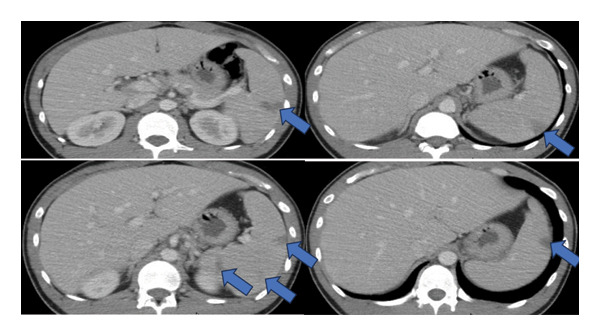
Still images from CT abdomen and pelvis from the patient’s first presentation to the referring hospital demonstrating splenic lesions, thought to be splenic infarcts.
